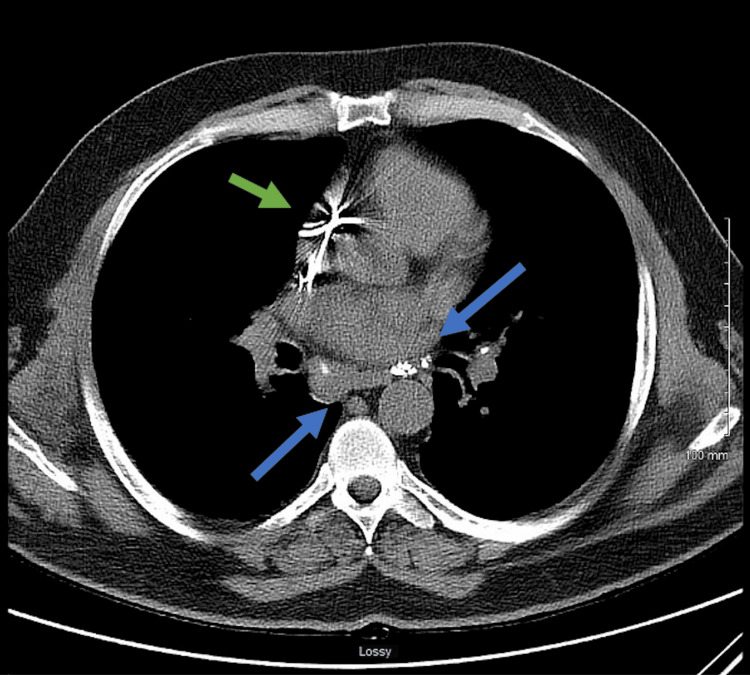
Non-contrast CT chest demonstrates lymphadenopathy, characterized by focal calcifications prominently within the subcarinal space and on both sides of the hilar regions, as indicated by the blue arrows. Defibrillator leads, with wires, are clearly visible within the right atrium and ventricle (green arrow).
